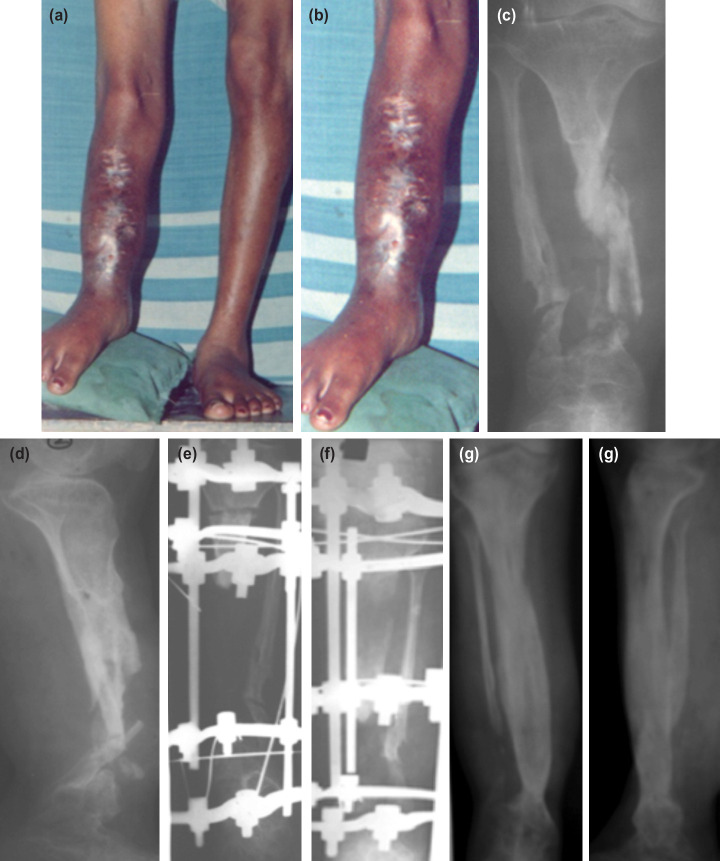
(a) Pre-op clinical photo of a case of infected non-union tibia with LLD. (b) Magnified image showing infected part with sinuses. (c) Pre-op AP view radiograph showing infected non-union. (d) Pre-op lateral view radiograph showing infected non-union. (e) After radical debridement and corticotomy with massive bone gap. (f) Subsequent radiograph during bone transport showing regenerate bone. (g) Final follow-up AP view radiograph showing union and regenerate. (h) Final follow-up Lat view radiographs showing union and regenerate.
